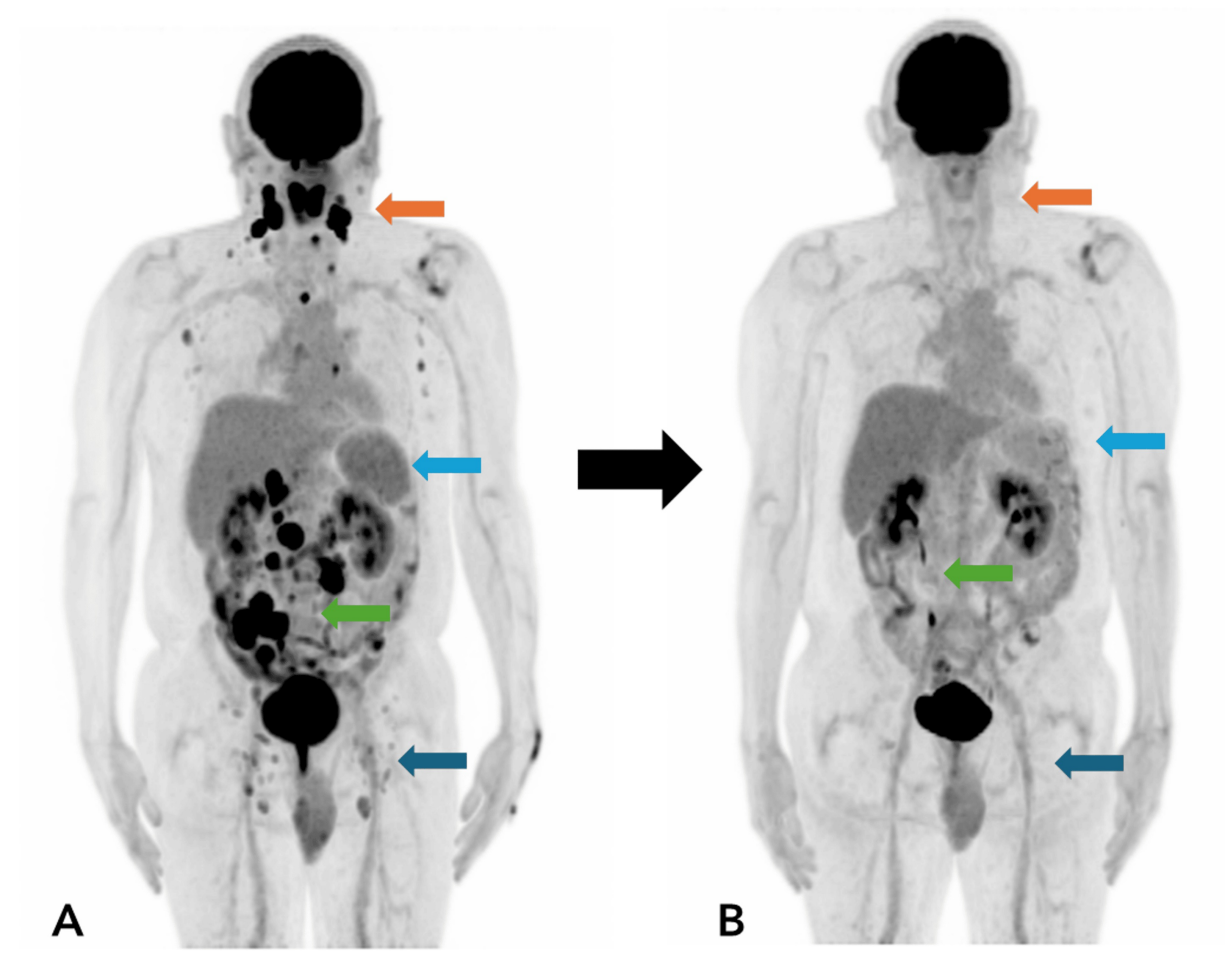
a) The maximum intensity projection (MIP) image from the FDG-PET/CT performed in November 2024 demonstrates intense hypermetabolic activity involving both the supradiaphragmatic and infradiaphragmatic lymph node regions, with additional extranodal involvement of osseous structures and intestinal segments. FDG-avid involvement of Waldeyer’s ring is indicated by the orange arrow. A reversal of the normal liver-to-spleen metabolic gradient is observed (light blue arrow). Asymmetric hypermetabolic thickening of the first portion of the duodenum and terminal ileum is noted (green arrow). Bilateral iliac chain and inguinal lymphadenopathy with increased FDG uptake is also evident (dark blue arrow). b) Follow-up MIP image from the FDG-PET/CT performed in May 2025, following completion of six cycles of R-CHOP chemotherapy, demonstrates complete or near-complete metabolic resolution of previously noted nodal and extranodal lesions. Residual uptake is below hepatic background levels. Arrows correspond to sites of prior disease involvement, now showing metabolic response consistent with the treatment effect.
